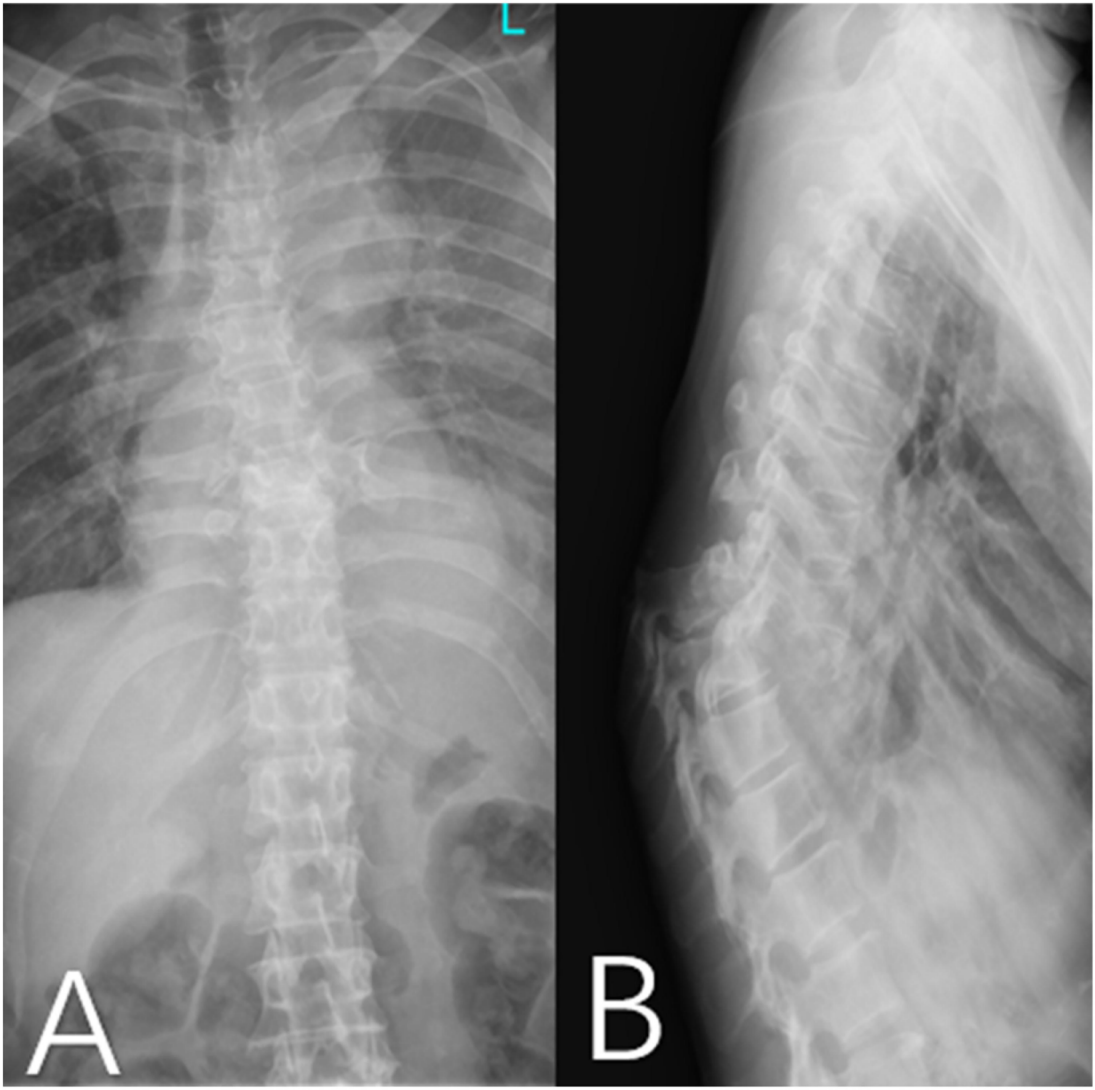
(A) Anteroposterior and (B) lateral radiographs of the thoracic spine demonstrate a fracture-dislocation at the T8-T9 segment. The distal fracture fragment is displaced posteriorly and superiorly, accompanied by a marked kyphotic deformity. Additionally, fractures of the T8 and T9 pedicles are evident, characterized by interruption and asymmetry of the pedicular contours, indicating severe disruption of the three-column spinal structure.
